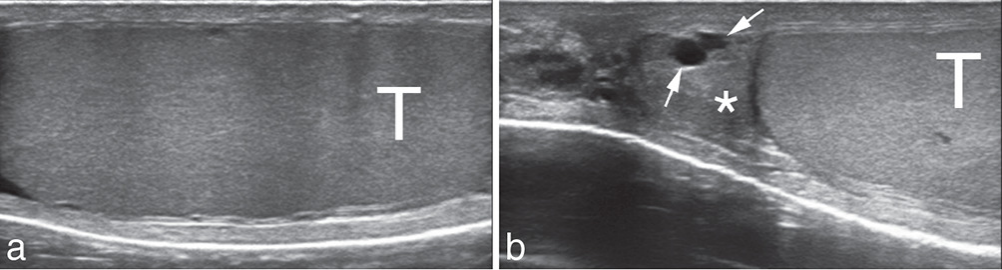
Normal appearance of (a) the left testis (T) and (b) the left epididymis (asterisk). Two small simple cysts are seen (arrows). Power Doppler evaluation (not shown) was unremarkable.
